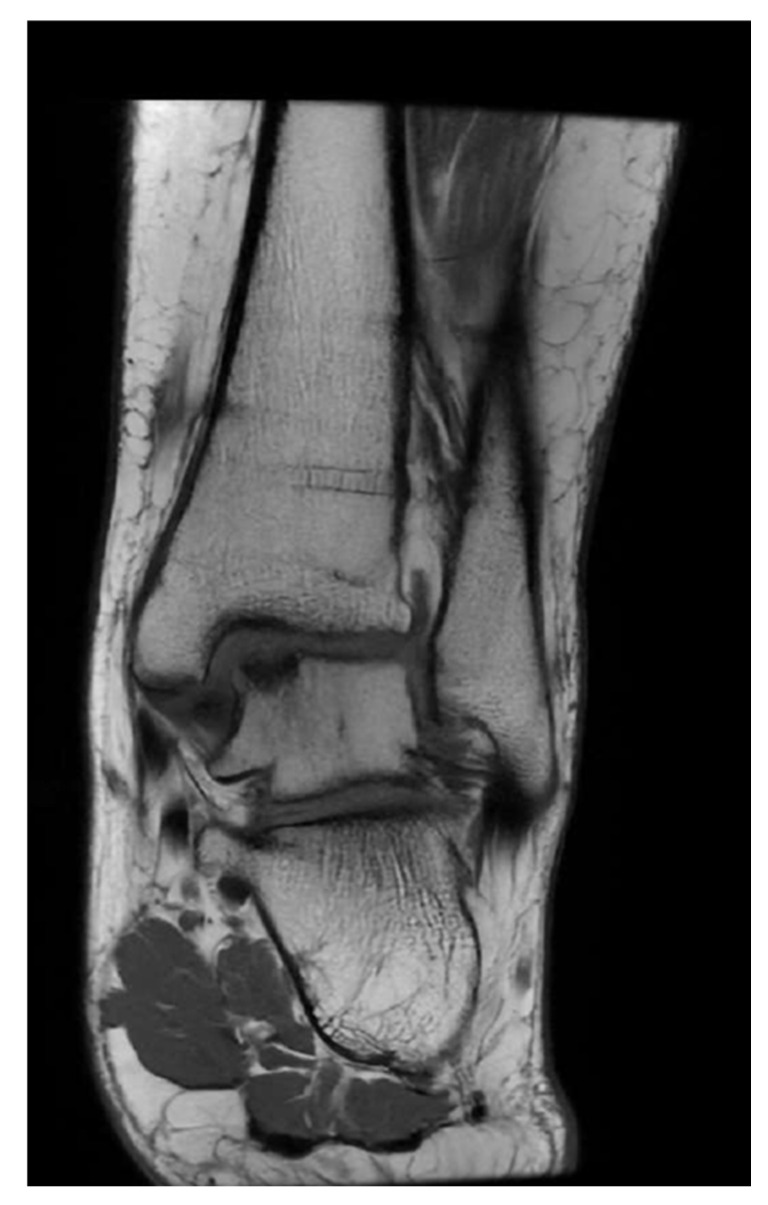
Representative MRI scan (coronal view, T1 sequence) of a medial osteochondral lesion of the talus prior to treatment with AutoCart™.
